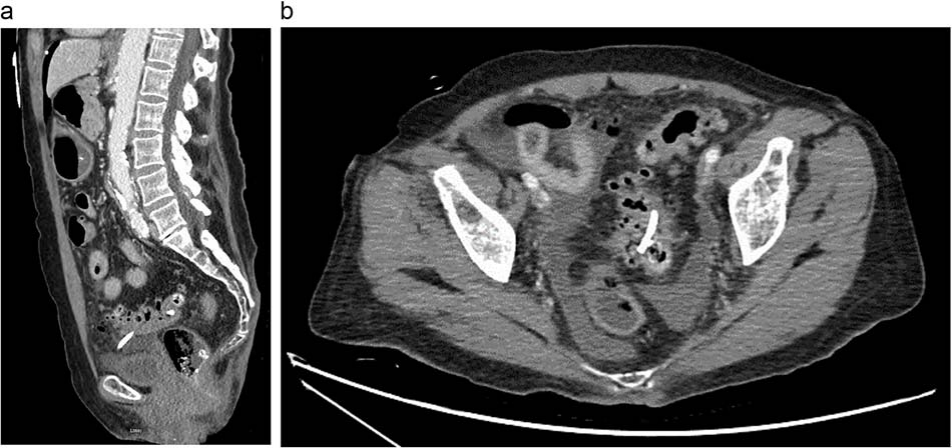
Abdominal CT showing migrated biliary stent that perforates the sigmoid colon in the sagittal (a) and axial (b) views. Pneumoperitoneum and free fluid in the pelvis are also noted.
